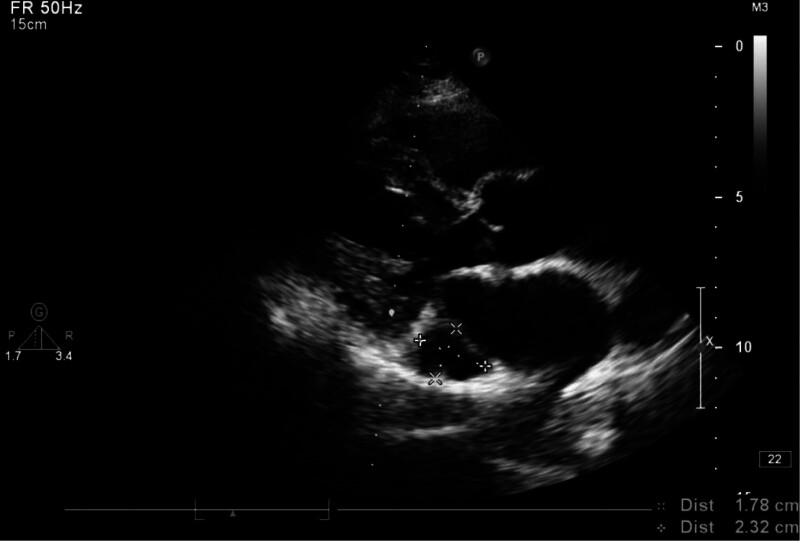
Echocardiogram shows coronary sinus dilatation.
